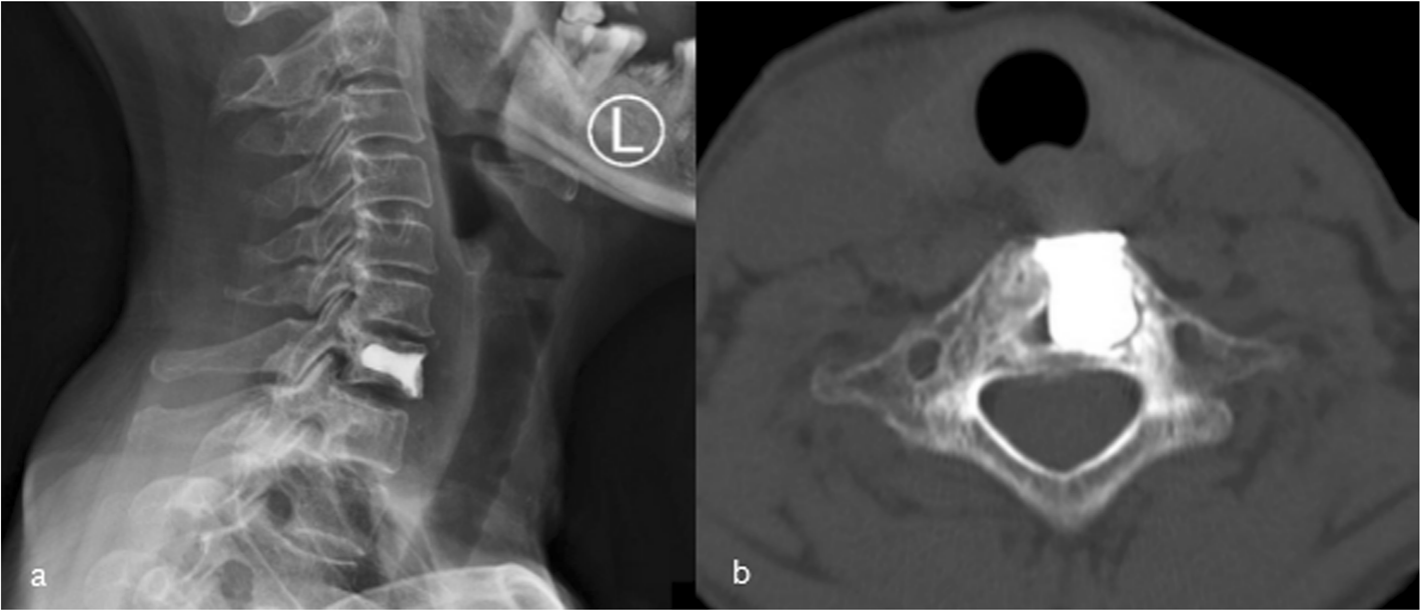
Three days after surgery imaging of the C7 vertebral body after cement filling (a, b): a X-ray, b CT
